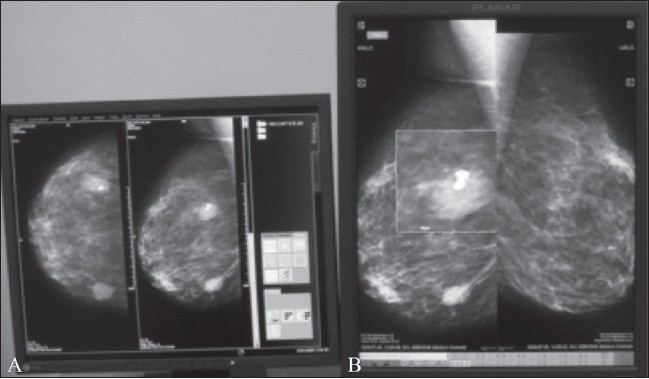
Landscape (A) versus portrait (B) mode. By virtue of being lightweight and thin, LCD monitors can be placed in a portrait mode that corresponds with the 17×14 format of images obtained in chest and skeletal radiography. Mammography images can be optimally viewed in the ‘portrait’ (B) rather than the ‘landscape’ mode (A), as shown here
